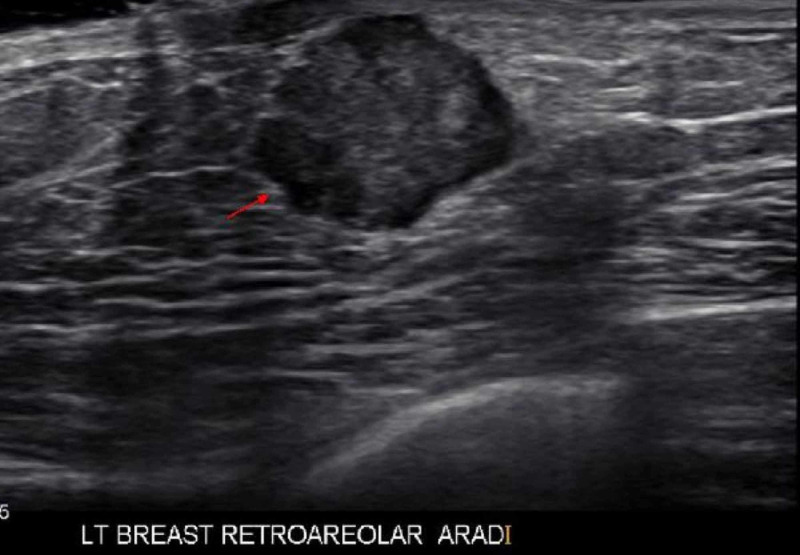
Ultrasound Left breast ultrasound showing hypoechoic vascular mass measuring 1.8 cm x 1.8 cm x 1.5 cm in size.
